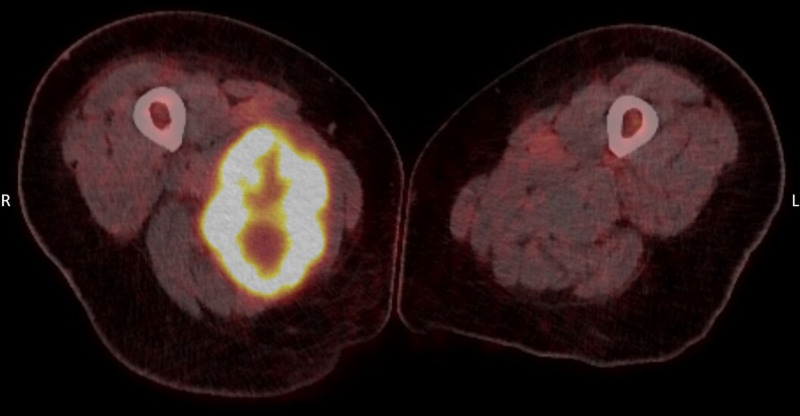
Fused FDG-PET/CT images showing a large, necrotic, FDG-avid metastasis in the upper right leg. The lesion has a largest diameter of 14.5 cm and is located near the abductor magnus muscle. CT, computed tomography; FDG, fluorodeoxyglucose.
